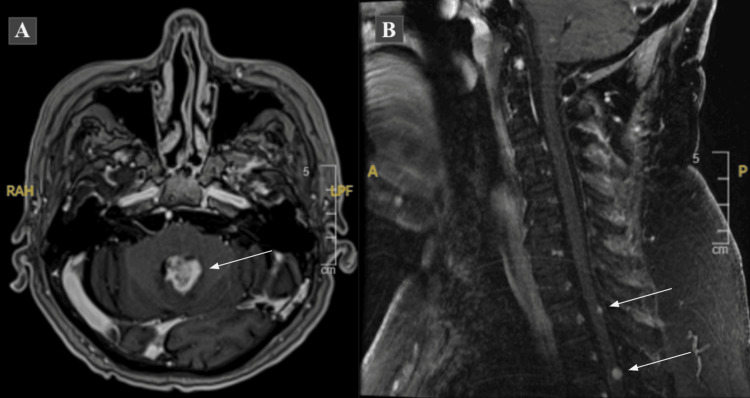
Leptomeningeal spread demonstrated on MRI Figure 1A: axial T1 post-contrast MRI of the brain demonstrating a heterogeneously enhancing mass occupying the fourth ventricle. Figure 1B: sagittal T2 FLAIR MRI of the cervical spine showing foci of nodular enhancement within the thoracic spine consistent with leptomeningeal spread FLAIR: fluid-attenuated inversion recovery; MRI: magnetic resonance imaging
